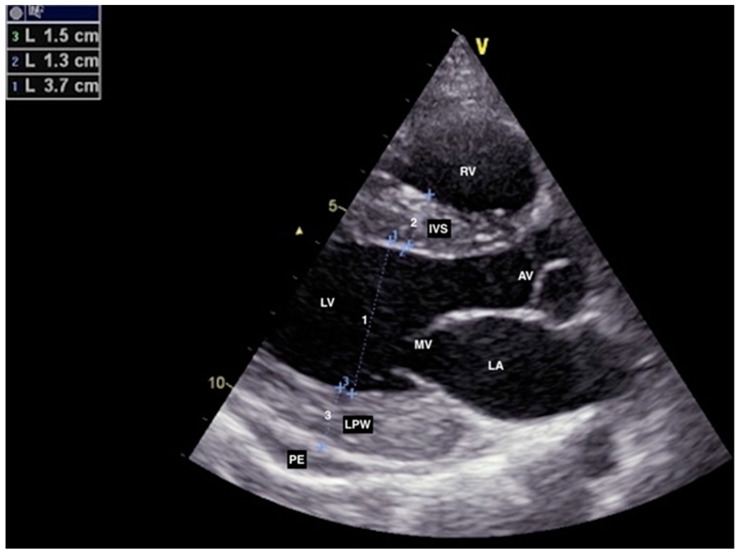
Second transthoracic echocardiography showing markedly increased thickness of left ventricle (LV) walls (IVS, LPW) in parasternal long axis (PLAX) view. AV: aortic valve, IVS: interventricular septum, LA: left atrium, LPW: left posterior wall, MV: mitral valve, PE: pericardial effusion, RV: right ventricle.
